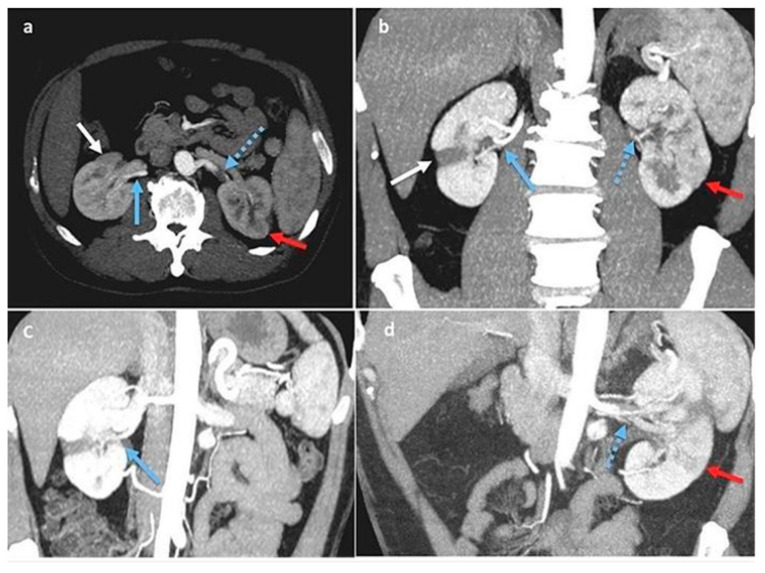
(a–d): Multiplanar reconstructions on Anglo-CT scan, arterial phase. Right kidney: focal ischemic area (white arrow) associated with sub-acute dissection of the inferior polar artery (blue arrow). Left kidney: acute dissection of renal artery (blue arrow with dots) with focal acute ischemic area (red arrow).
